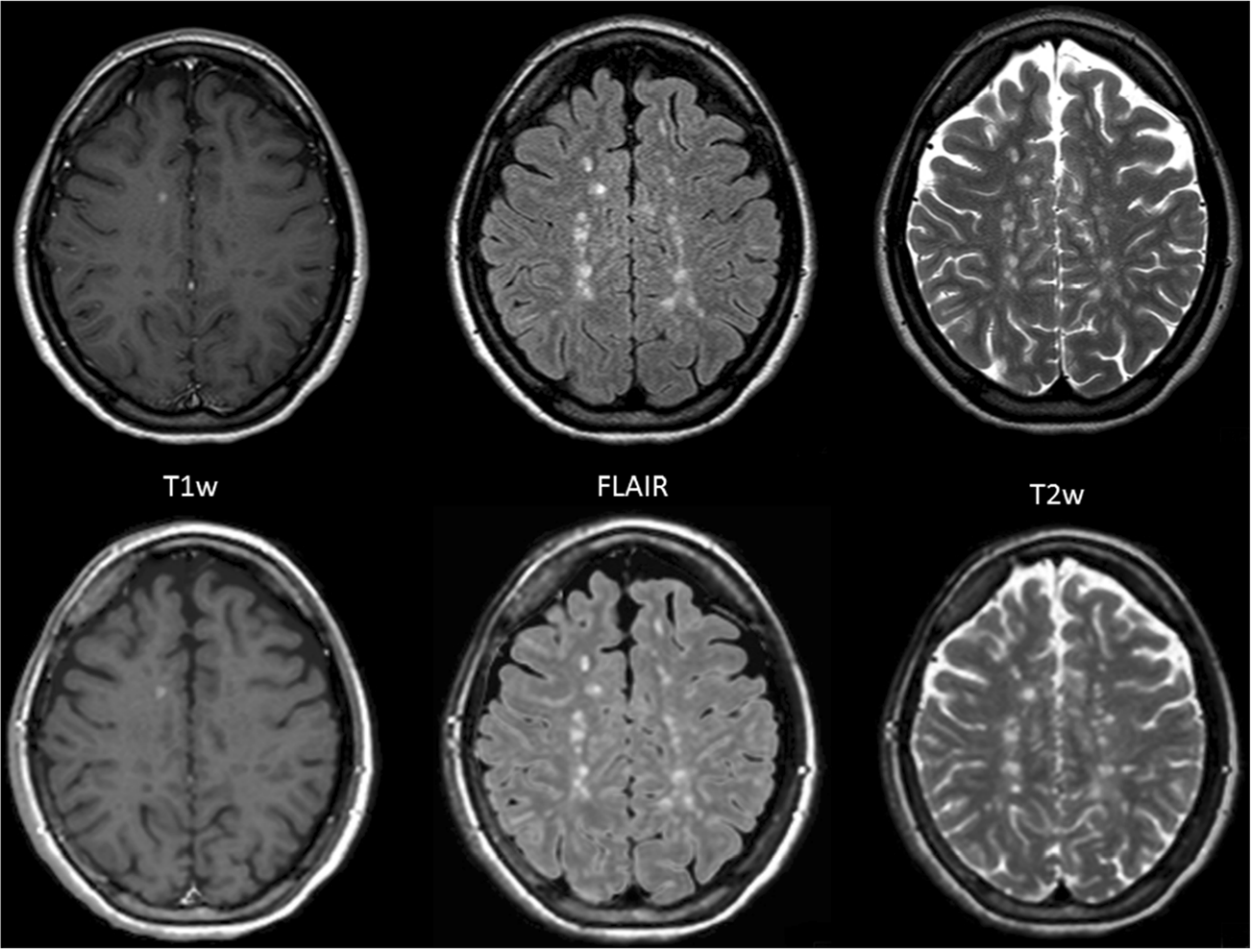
Example of conventional images (*upper row*) and synthetic T1W (contrast-enhanced), T2W, and FLAIR images (*lower row*) in an MS patient
